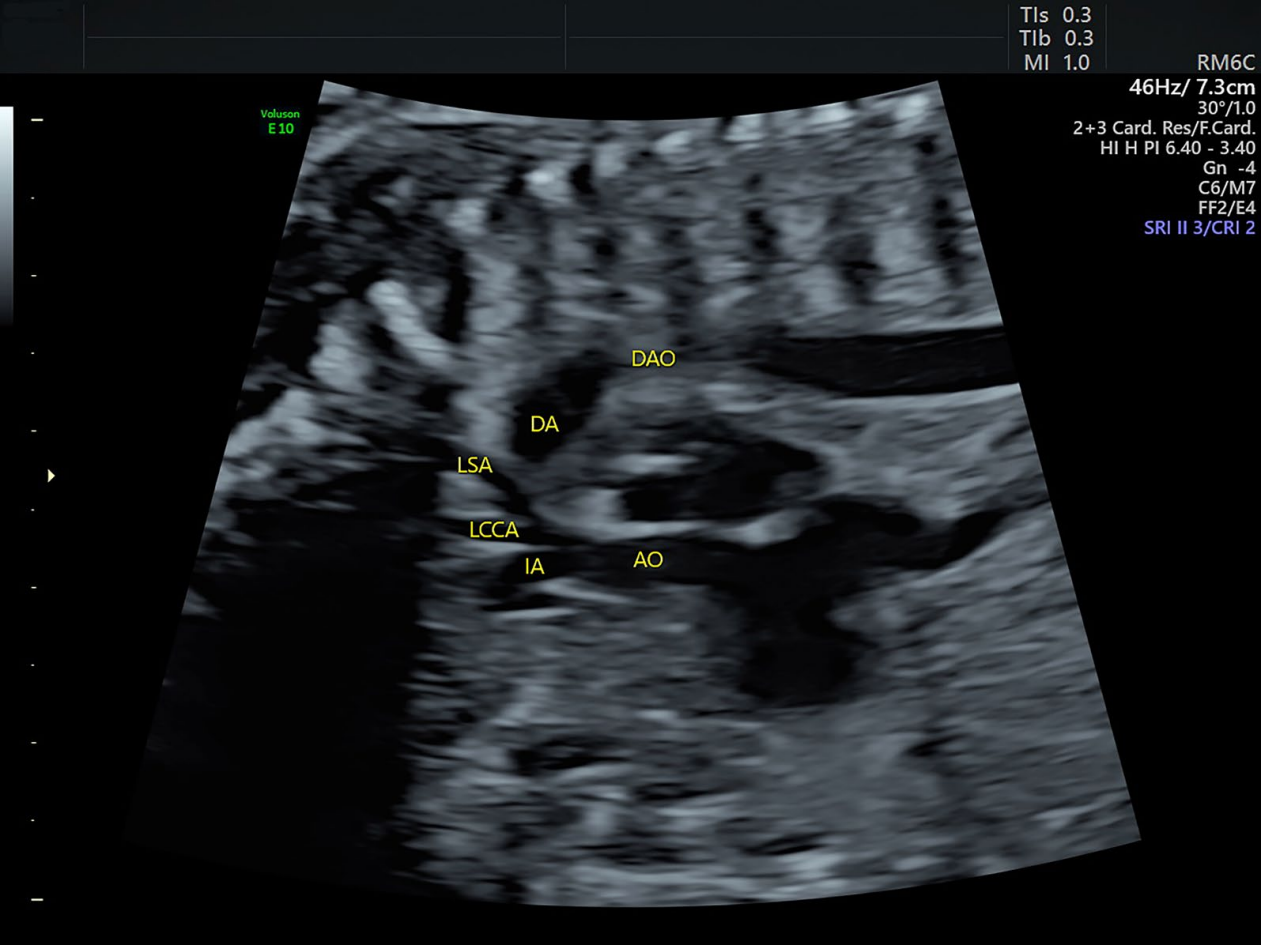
Sonograms of the fetus of coarctation of the aorta at the sagittal view. The sagittal view shows that the ascending aorta goes straight upward and gives off three brachiocephalic arteries while the aortic arch does not appear. AO: aorta; DA: ductus arteriosus; DAO: descending aorta; IA: innominate artery; LCCA: left common carotid artery; LSA: left subclavian artery
